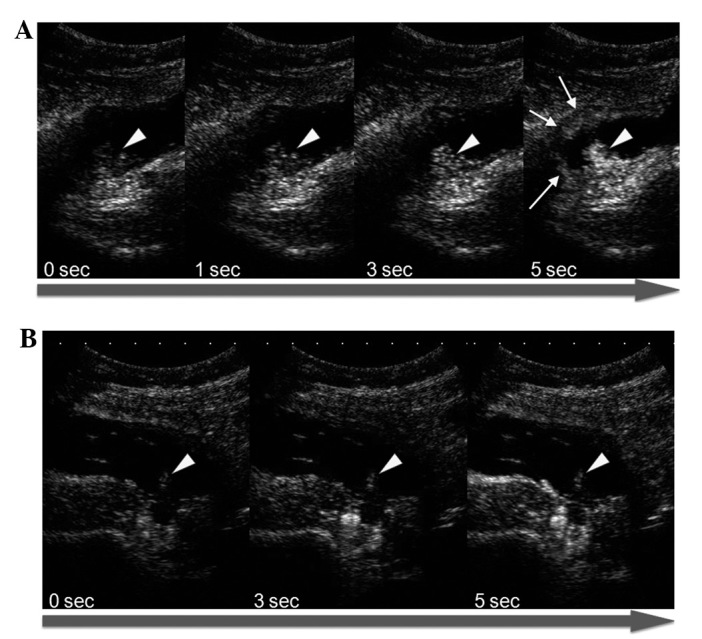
Abdominal contrast-enhanced ultrasonograms obtained in August 2012. A mass was visible from (A) the appendicular ostium and (B) from the appendicular tip. The mass wall (A, arrow) and the projection (A, arrowhead) on the appendicular ostium were enhanced within 5 sec of contrast agent arrival, whereas no enhancement was visible in the projection on the appendicular tip (B, arrow head).
